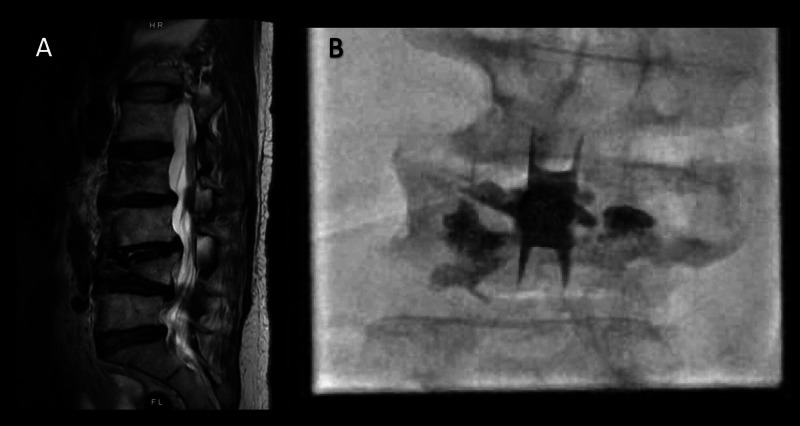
(A) Sagittal T2-weighted MRI image demonstrates vertebral compression fractures as well as multilevel discogenic and degenerative disease, worst at L3-L4. (B) Intraprocedural AP radiograph demonstrates successful placement of the superion interspinous spacer device at L3-L4. PMMA from prior augmentation is present at L4. AP, anteroposterior; PMMA, polymethyl methacrylate
